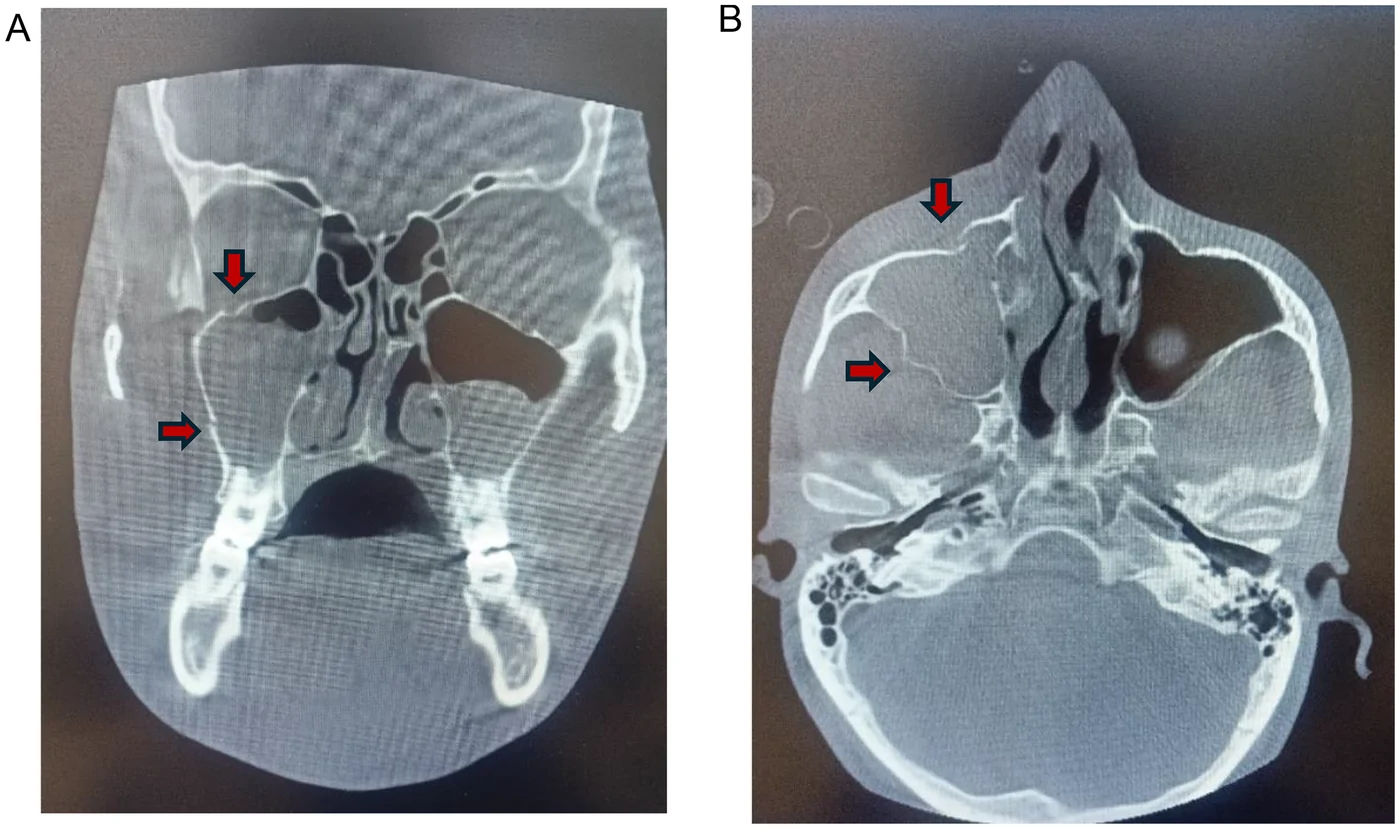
(A) immediate postoperative CT scan (coronal view). (B) Immediate postoperative CT scan (axial view).
